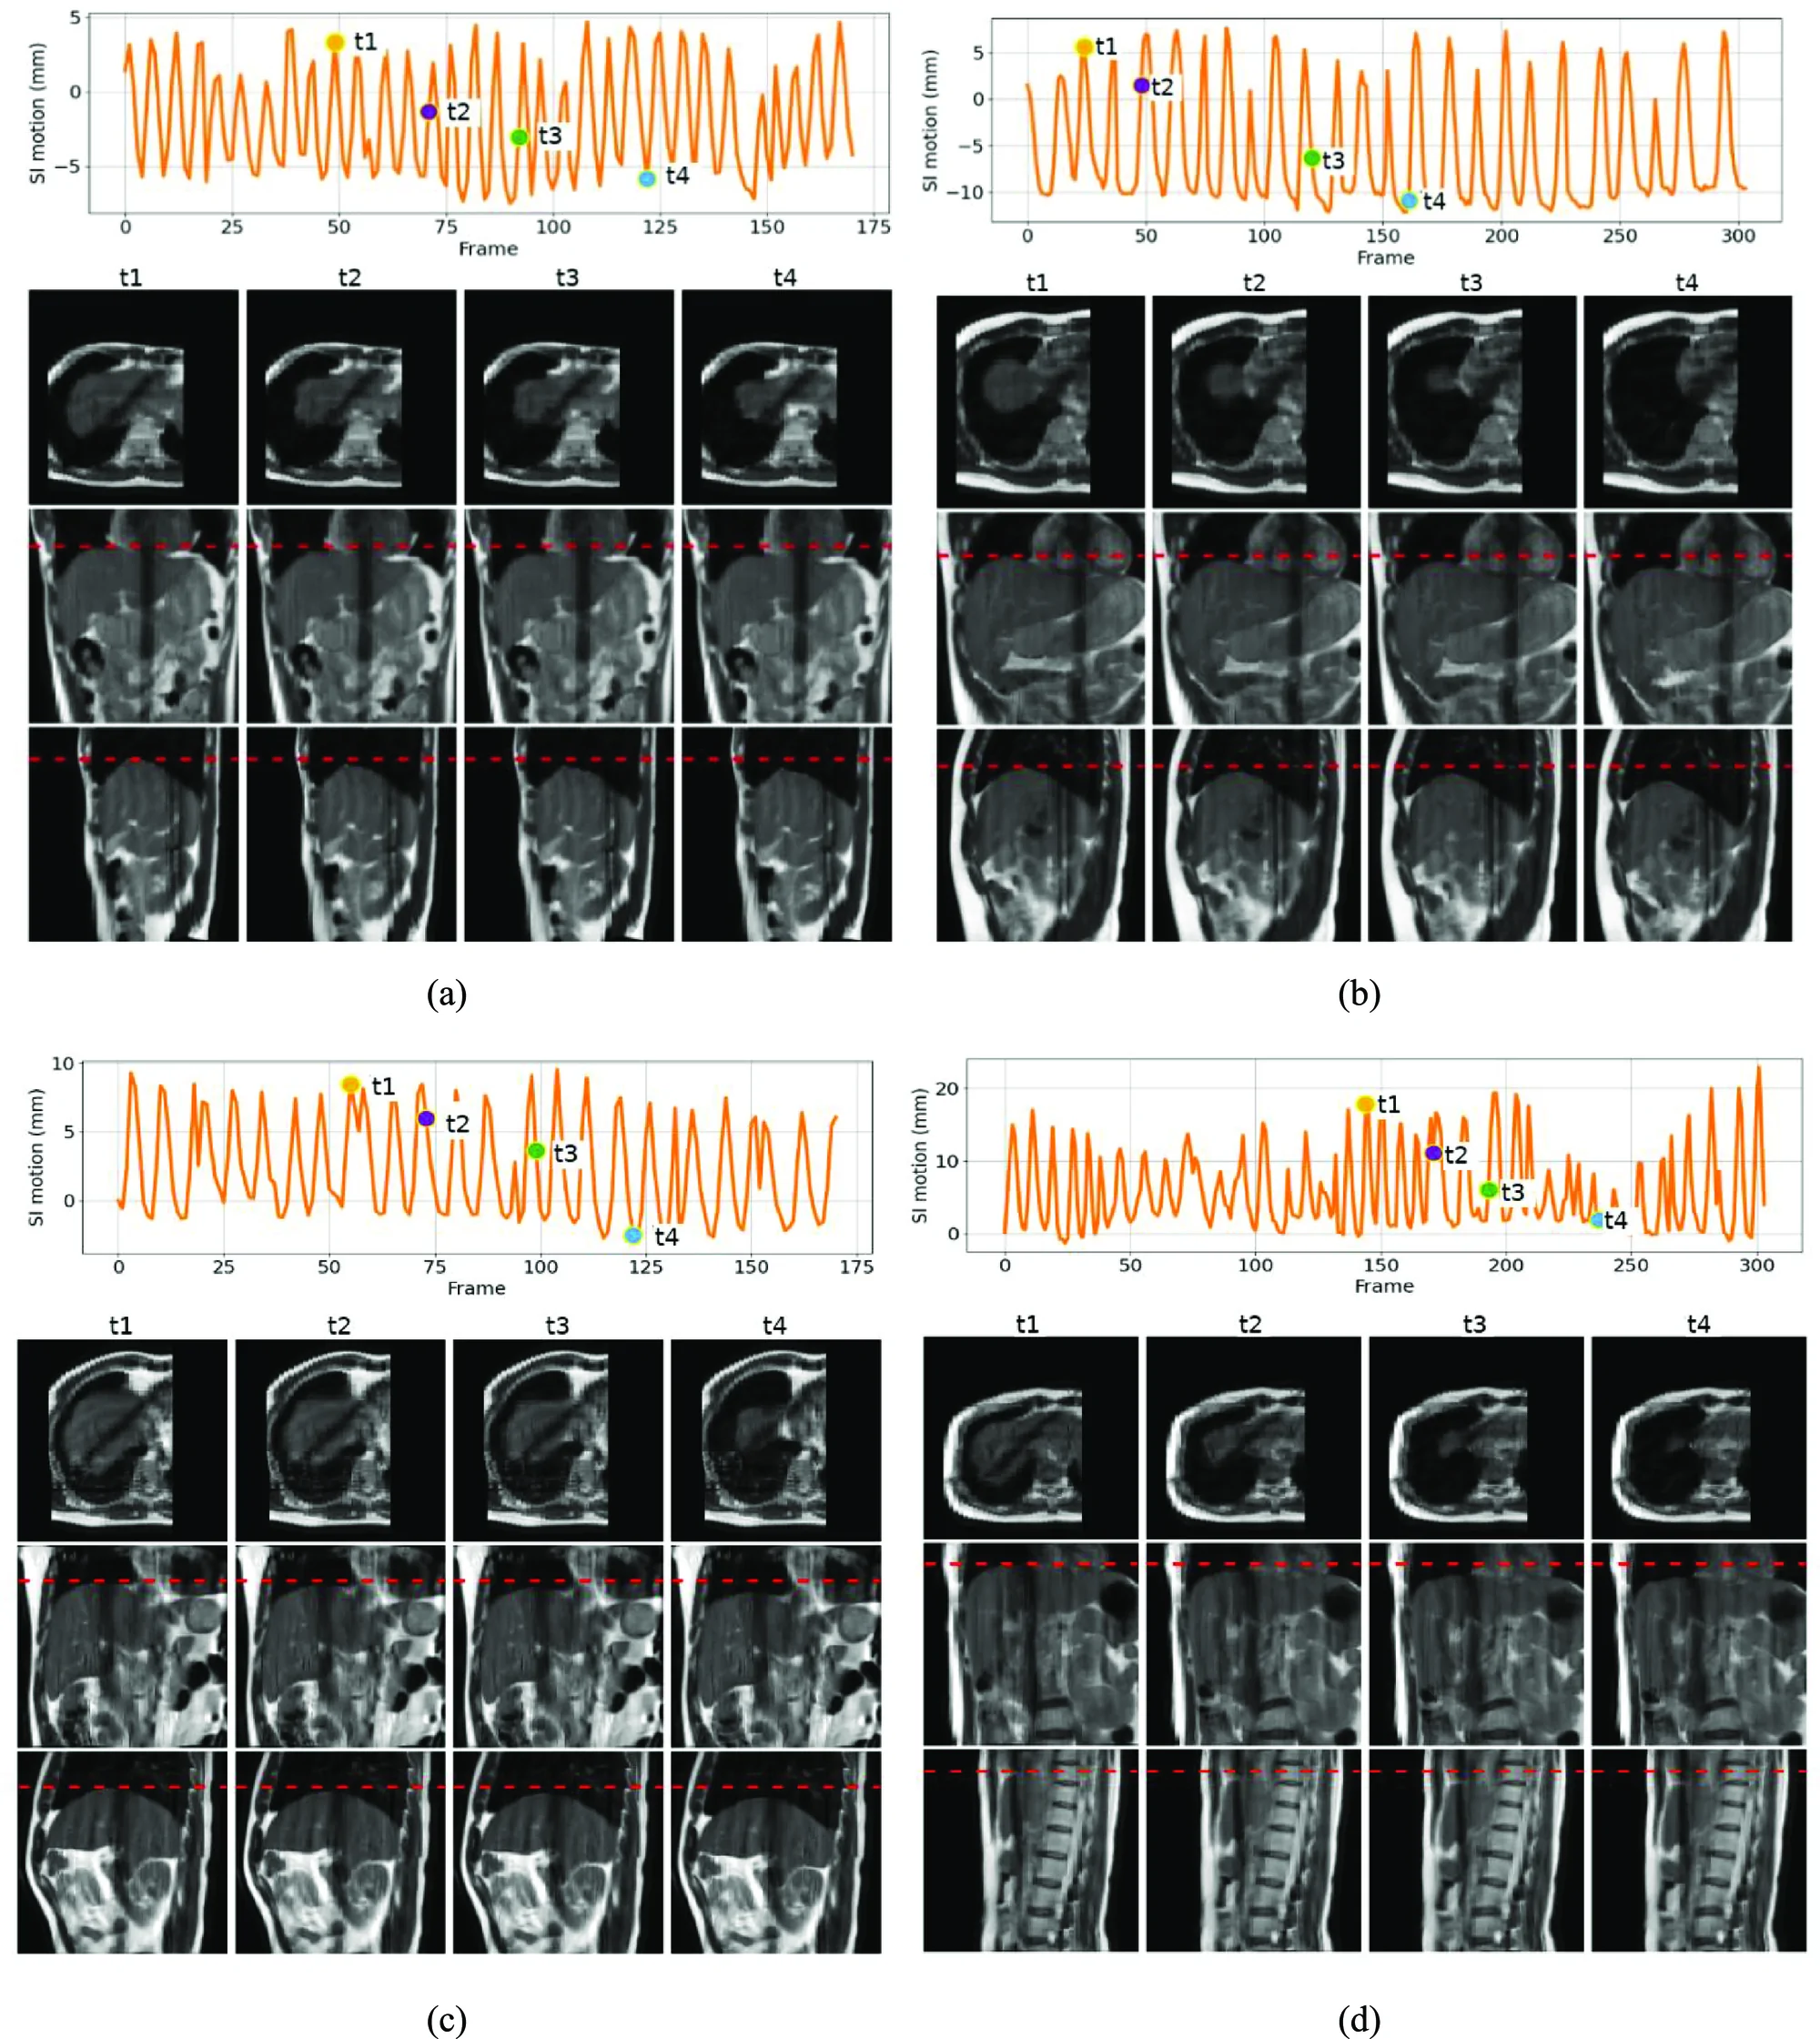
Time-resolved dynamic MR images of the four volunteers, (a)–(d). The first row shows the estimated liver center-of-mass trajectory along the SI direction. The subsequent rows display the reconstructed MR images in the axial, coronal, and sagittal views at four representative time points (*t*1–*t*4).
